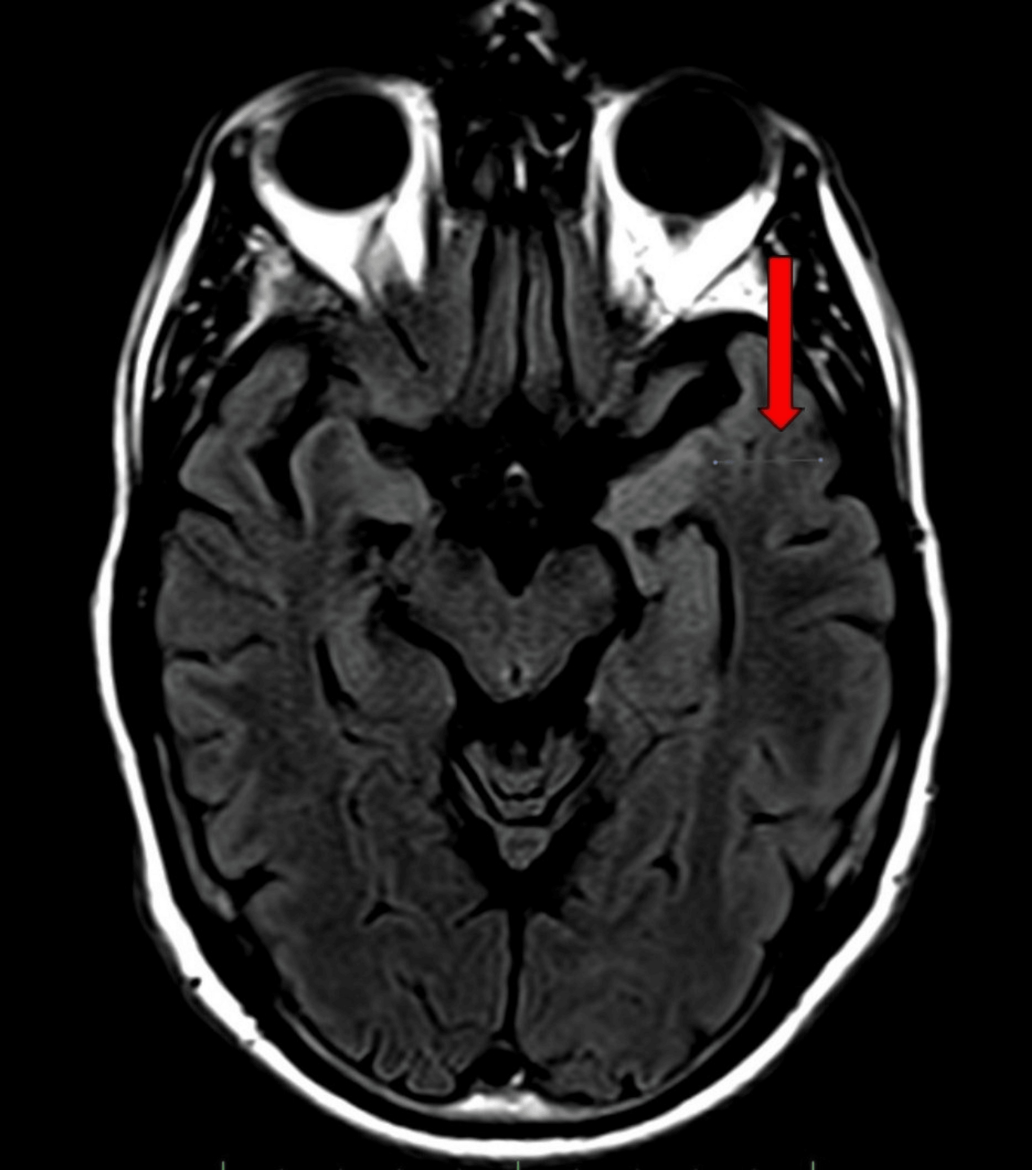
Diffusion weighted MRI imaging showing T2-flair in the left anteromedial temporal lobe, highlighted by the red arrow
